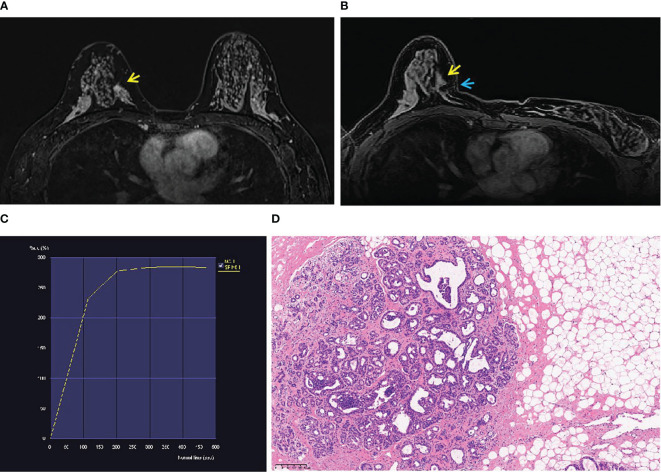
A 46-year-old woman’s physical examination. (A) A non-mass enhancement (yellow arrow) located in the inner quadrant of the right breast (BI-RADS 4A) was shown in T1-weighted fat-suppressed contrast-enhanced MRI. It was not detected by ultrasound or mammography. (B) The lesion (yellow arrow) was performed by MR-guided wire localization (blue arrow). (C) The dynamic contrast-enhanced time–signal intensity curve (TIC) appeared as “fast-plateau type”. (D) Postoperative pathological diagnosis was atypical hyperplasia.
